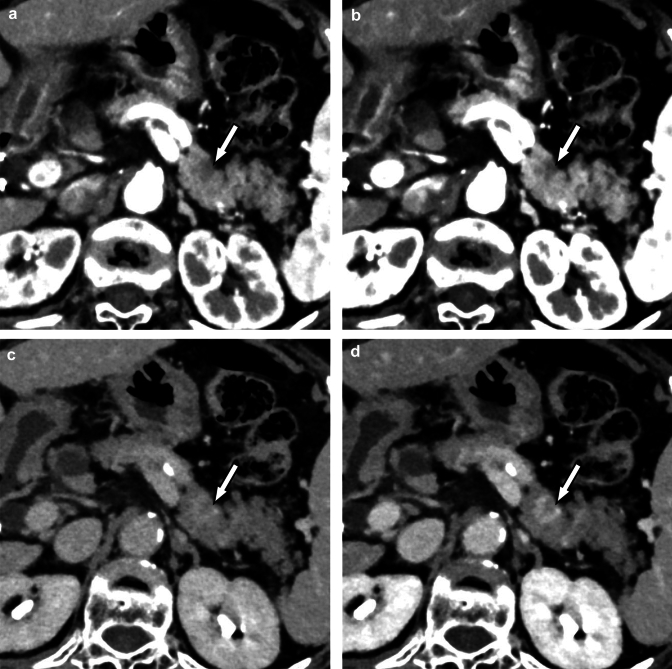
Pancreatic cancer is shown as a small hypovascular nodule in the tail of the pancreas (arrows) on the pancreatic parenchymal phase (arterial phase) photon-counting CT images at 70 (A) and 50 keV (B). Meanwhile, enhancement of the tumor (arrows) is shown during the equilibrium phase at 70 (C) and 50 keV (D). The delayed enhancement is more evident in the 50 keV image (D) than in the 70 keV image (C). Small pancreatic cancers are often not visualized in the pancreatic parenchymal phase but are visualized as delayed-enhancement lesions only in the equilibrium phase. Low-keV images obtained with photon-counting CT may help in the early detection of pancreatic cancer
